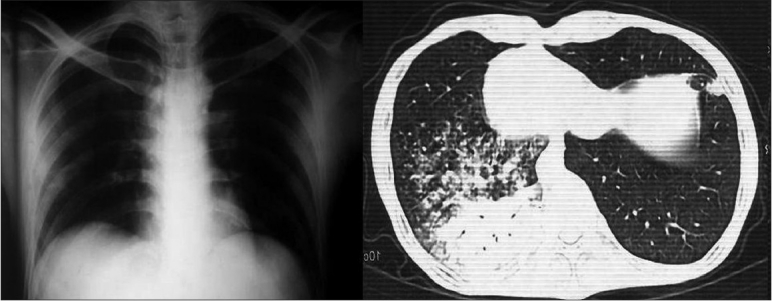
Normal chest x-ray but CECT revealing consolidation
